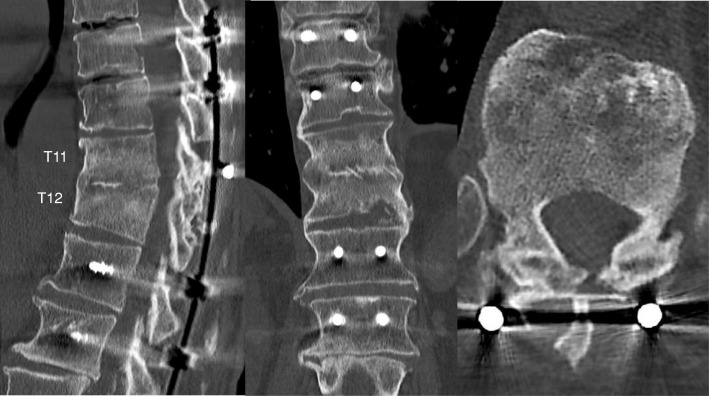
Two years after the operation, computed tomography demonstrated bone fusion at T11 and T12
